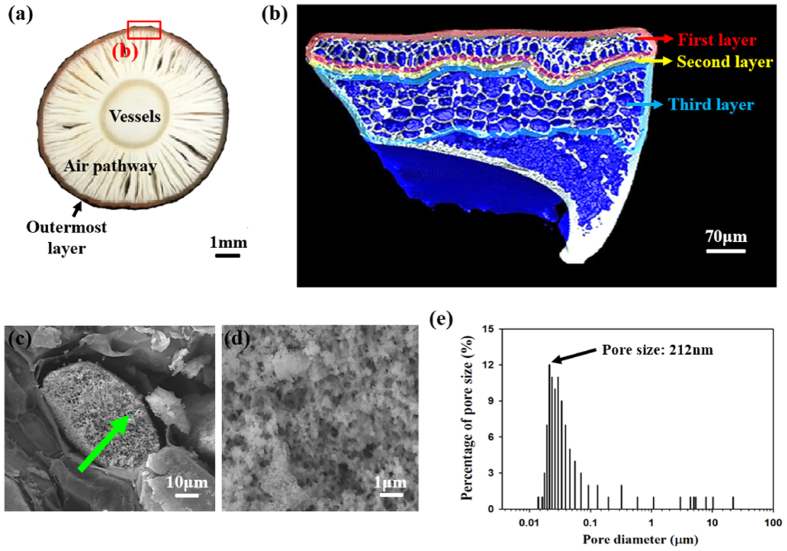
Triple-layered porous structure of RS roots. (a) Cross-sectional image of a mangrove root, exhibiting the outermost layer, air pathway, and vessels. (b) Reconstructed X-ray image shows the internal morphological structure of the outermost root layer. The outermost layer consists of three parts with different morphologies and functions marked as red region (first layer), yellow region (second layer), and blue region (third layer). (c) The second layer contains a porous membrane, whose pore size is in a scale of hundreds of nanometers. (d) Magnified view of the membrane, indicated by a green arrow in (c). Nanometer scale pores are irregularly distributed with various structures like conventional membrane filter. (e) Percentage distribution of pore-size measured by a mercury porosimetry. Pores of the membrane in the second layer are mainly at hundreds of nanometers in size. Size of 212 nm pores indicated by black arrow are the most.
